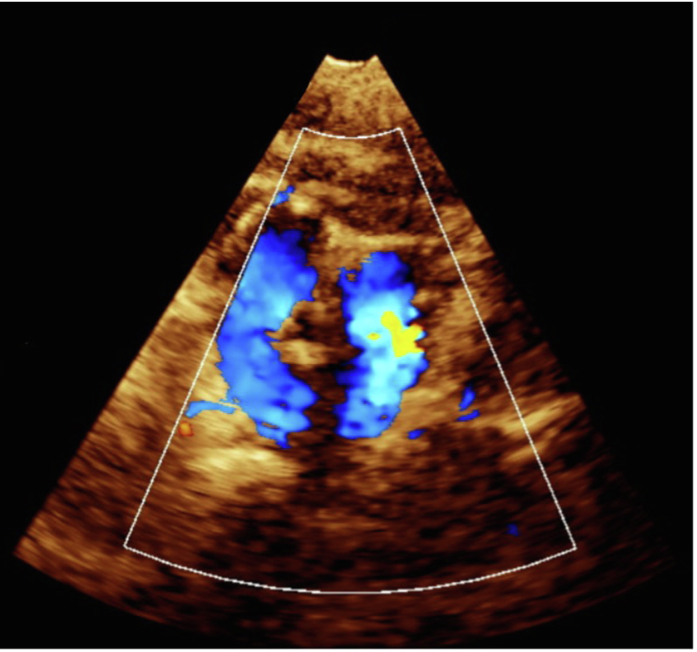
Transthoracic Echocardiography: Suprasternal Short-Axis View With Color Doppler, Demonstrating 2 Aortic Arch Limbs Encircling the Tracheal Air Column
